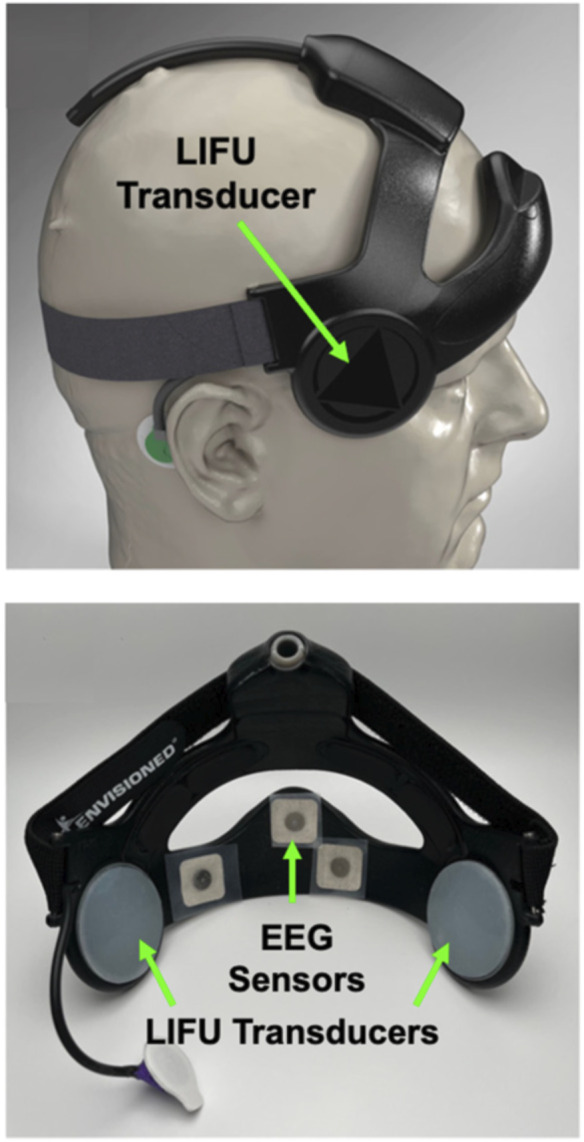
Wearable transcranial focused ultrasound neuromodulation devices. Recent breakthroughs in human factors, mechanical, materials, biomedical, and electrical engineering have enabled the development of wearable devices for neuromodulation by low-intensity transcranial focused ultrasound (LIFU). The device shown (Attune Neuroscience, Inc.) utilizes LIFU transducers to target different regions of the human brain and integrates electroencephalography (EEG) sensors for measuring brain wave activity patterns from the prefrontal cortex. This type of wearable device for neuromodulation by LIFU opens the possibility of developing closed-loop applications for human-in-the-loop control of robotic and semi-autonomous systems. Figure adapted from Reference (Meads et al., 2024).
